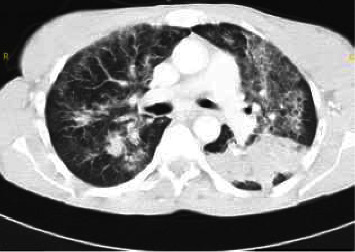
Chest computed tomography of Case 2: diffuse bilateral infiltrates mainly alveolar.
